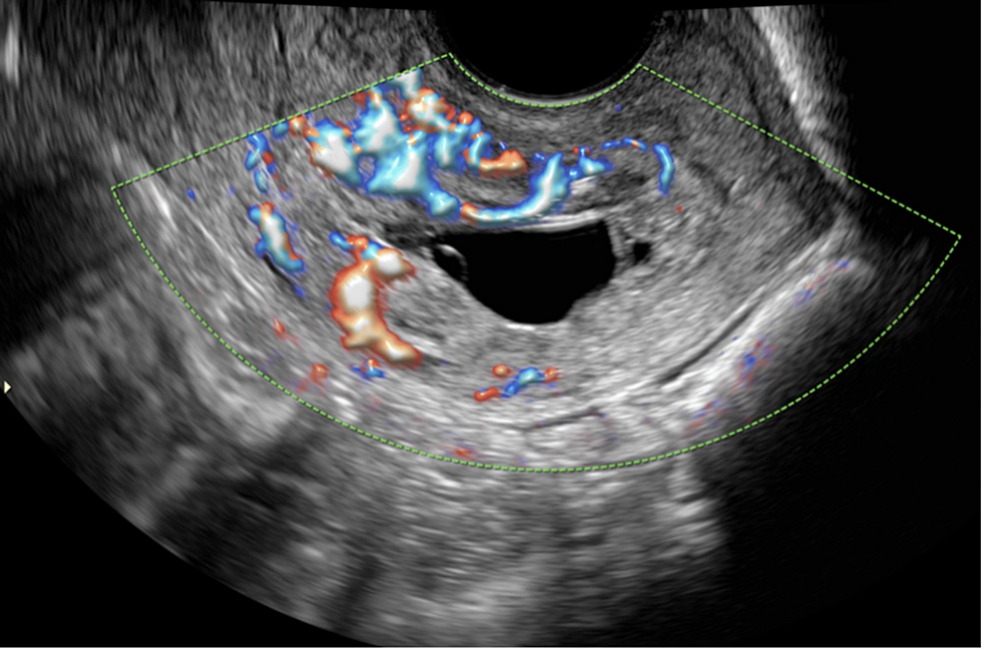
Transvaginal pelvic ultrasound at representation with trophoblastic tissue at the cervix and marked vascularity.
